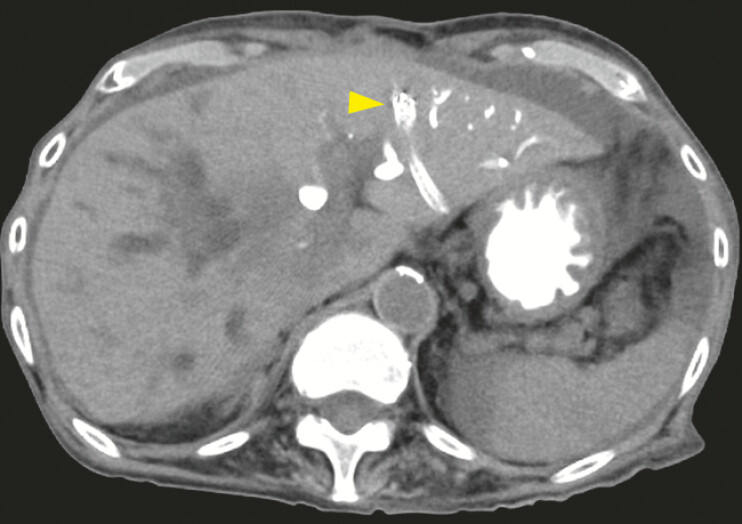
Computed tomography after endoscopic treatment. Misplacement of the initial metal stent into the hepatic parenchyma (yellow arrow head).
